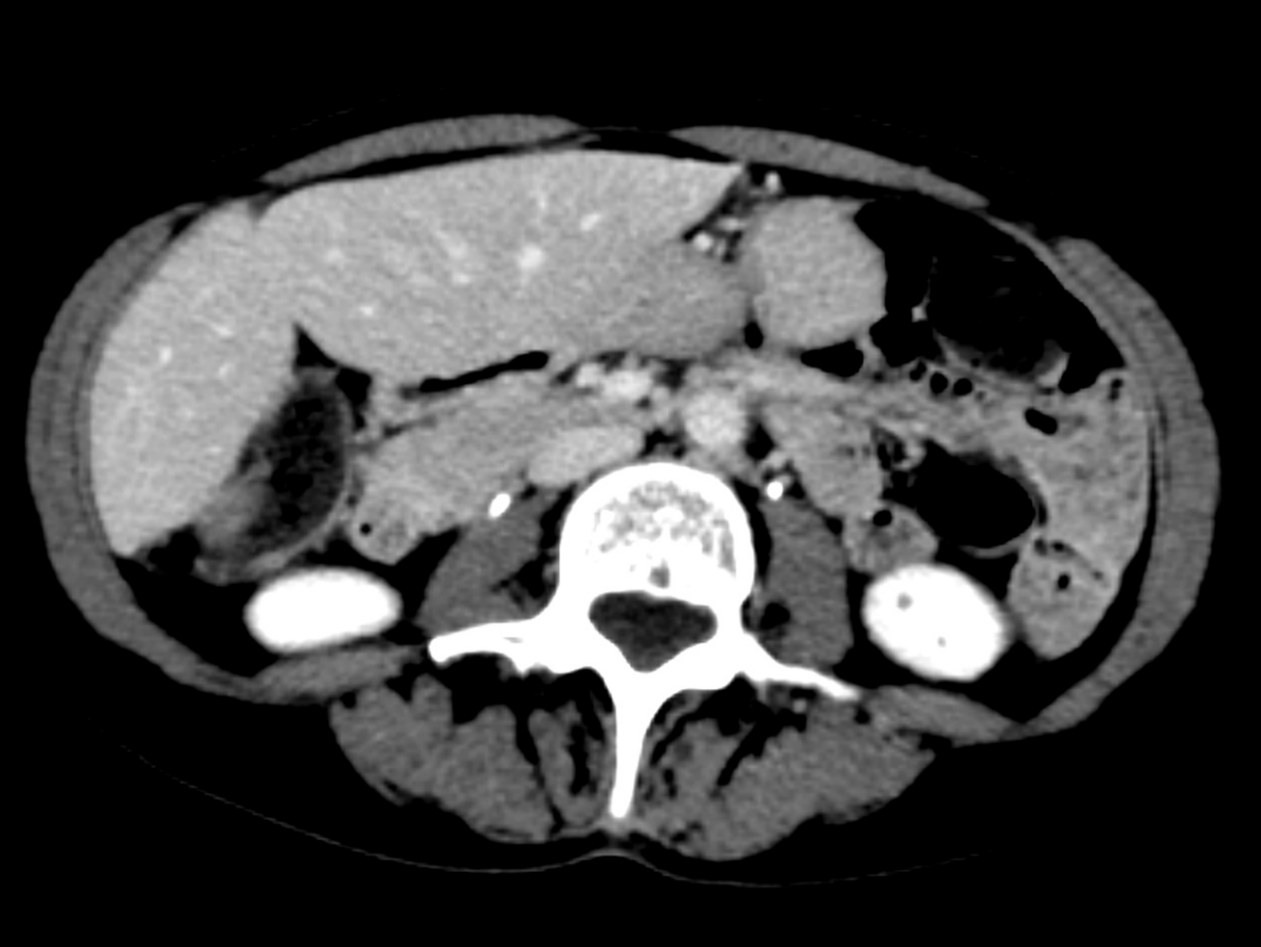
Representative axial slice from CT obtained nine months after second SIRT procedure
